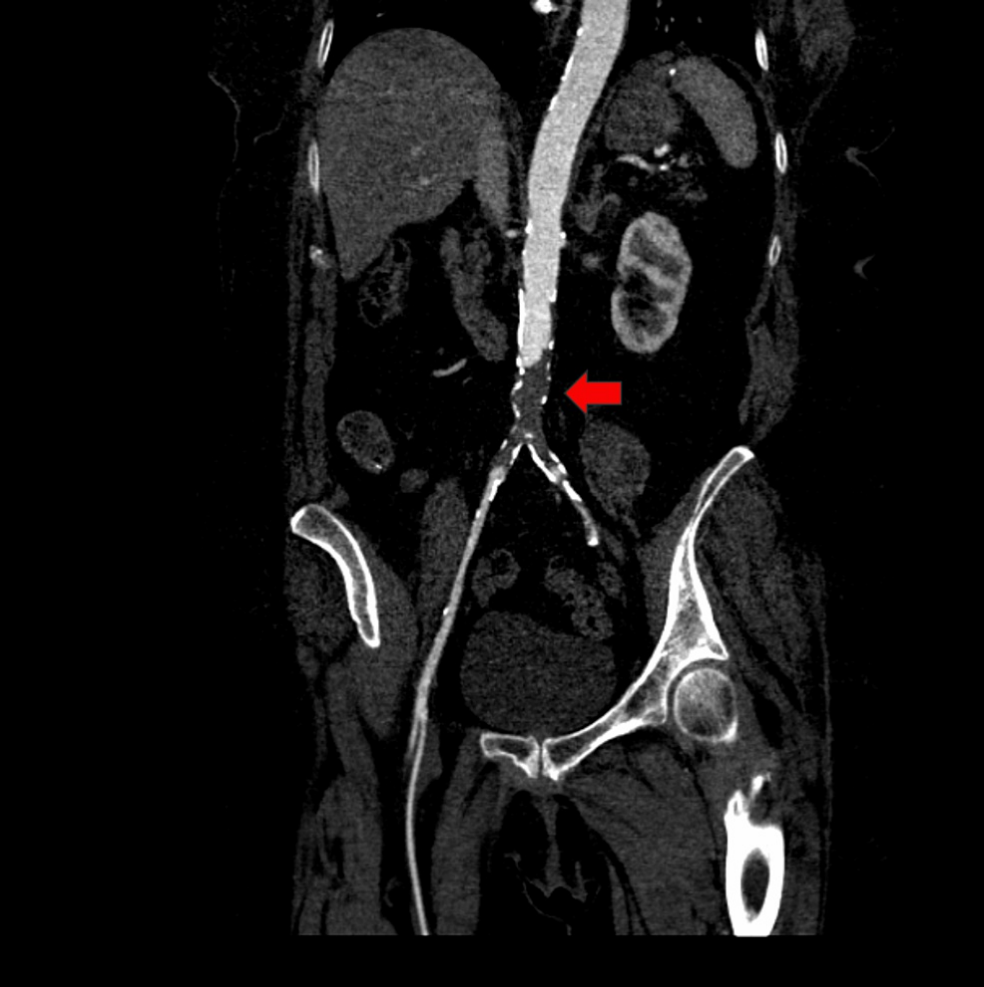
Computed tomography with angiography of the abdomen and pelvis showing occlusion of the infrarenal abdominal aorta and right and left common iliac arteries (red arrow)
